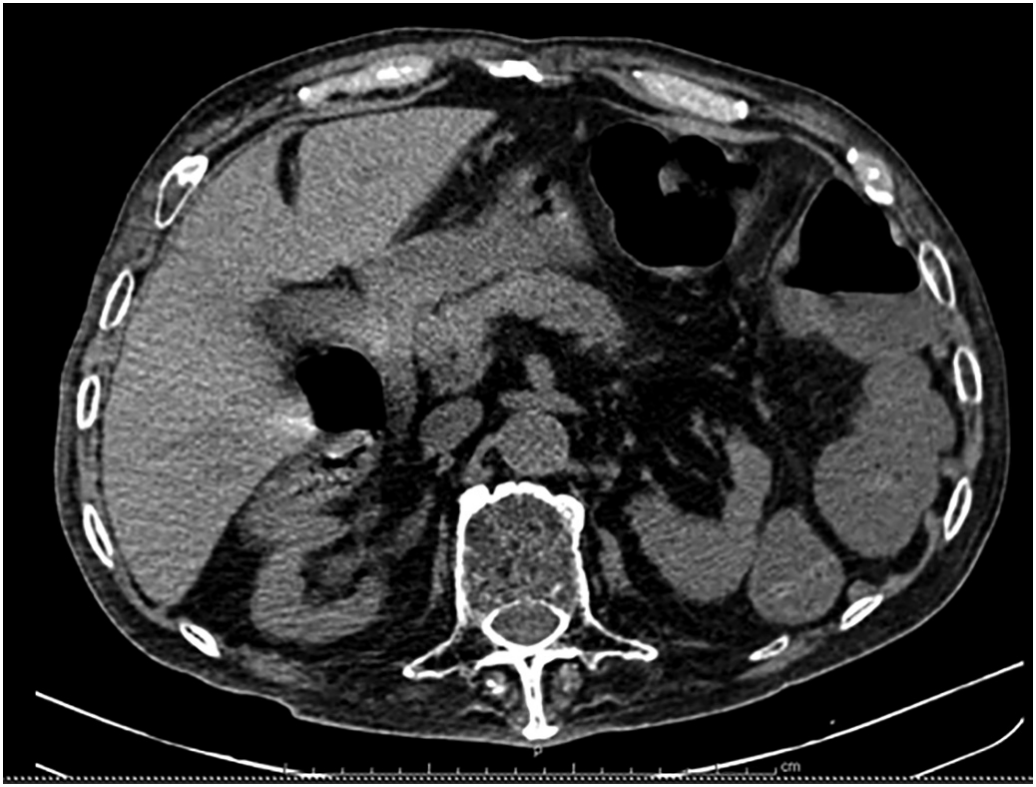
A preoperative non-contrasted computed tomography showing mild right sided hydronephrosis.
